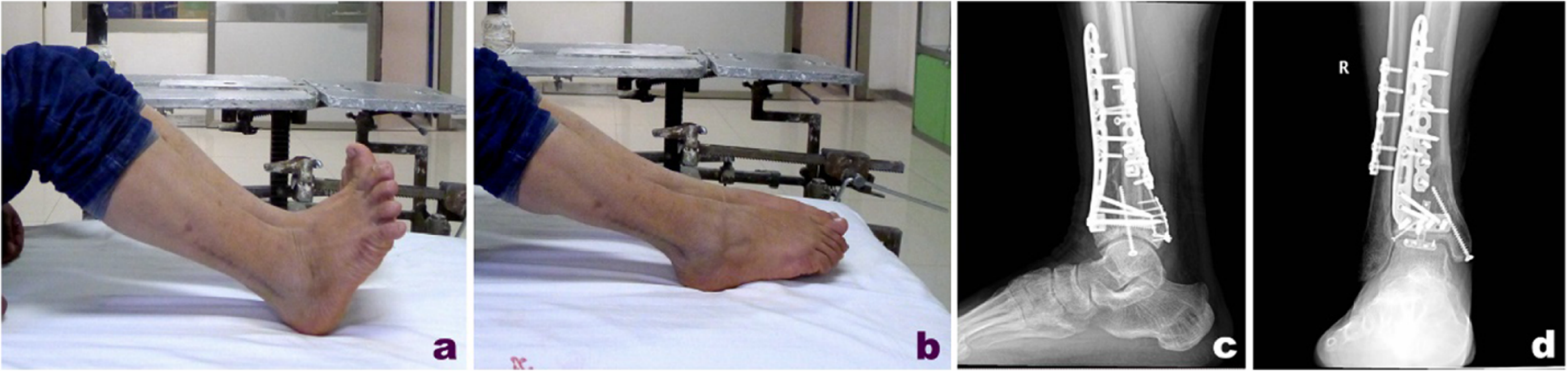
At 36 months follow-up, X-ray reexamination showed that the fracture healed well, no loosening or breakage of the internal fixation were observed, and function recovered well (a–d)
